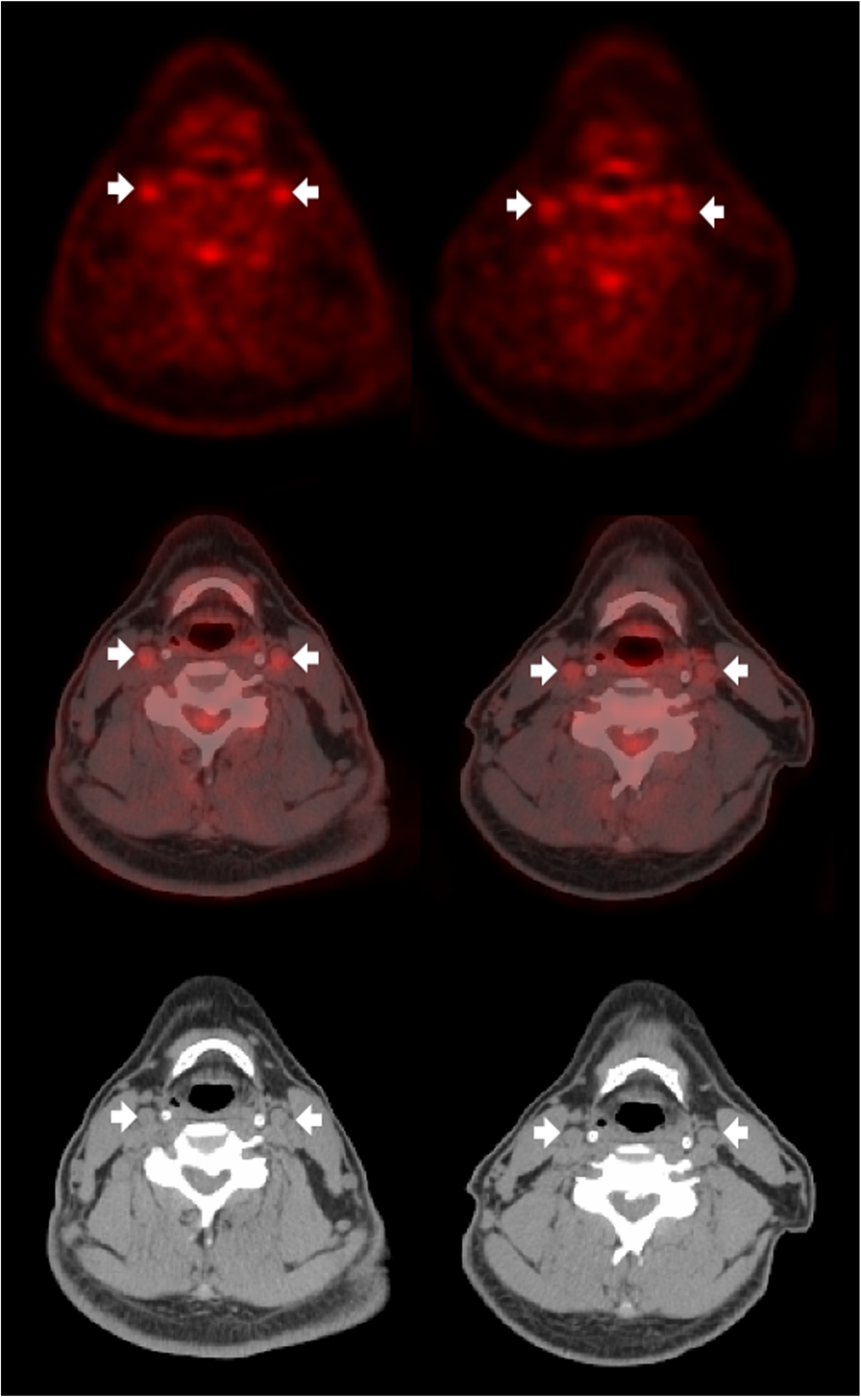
^18^FDG uptakes of the index vessel in a patient treated with ezetimibe/simvastatin (arrows). Representative CT (top), ^18^FDG-PET (middle), and ^18^FDG-PET/CT (bottom) images at baseline (left) and at the 6-month follow-up (right) are shown. ^18^FDG uptakes markedly decreased at the 6-month follow-up
